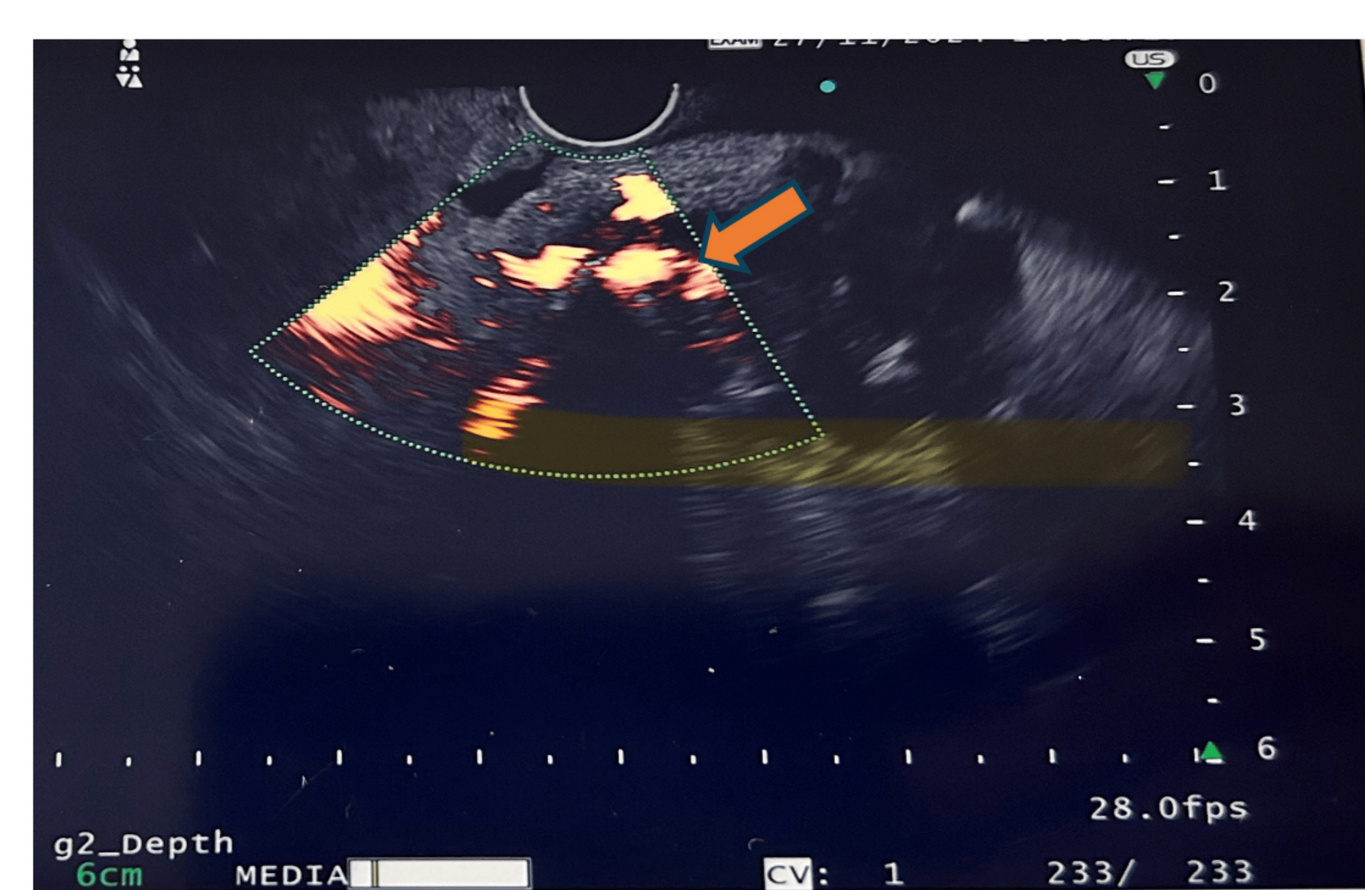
Color Doppler imaging showing blood flow inside the pseudoaneurysm.
